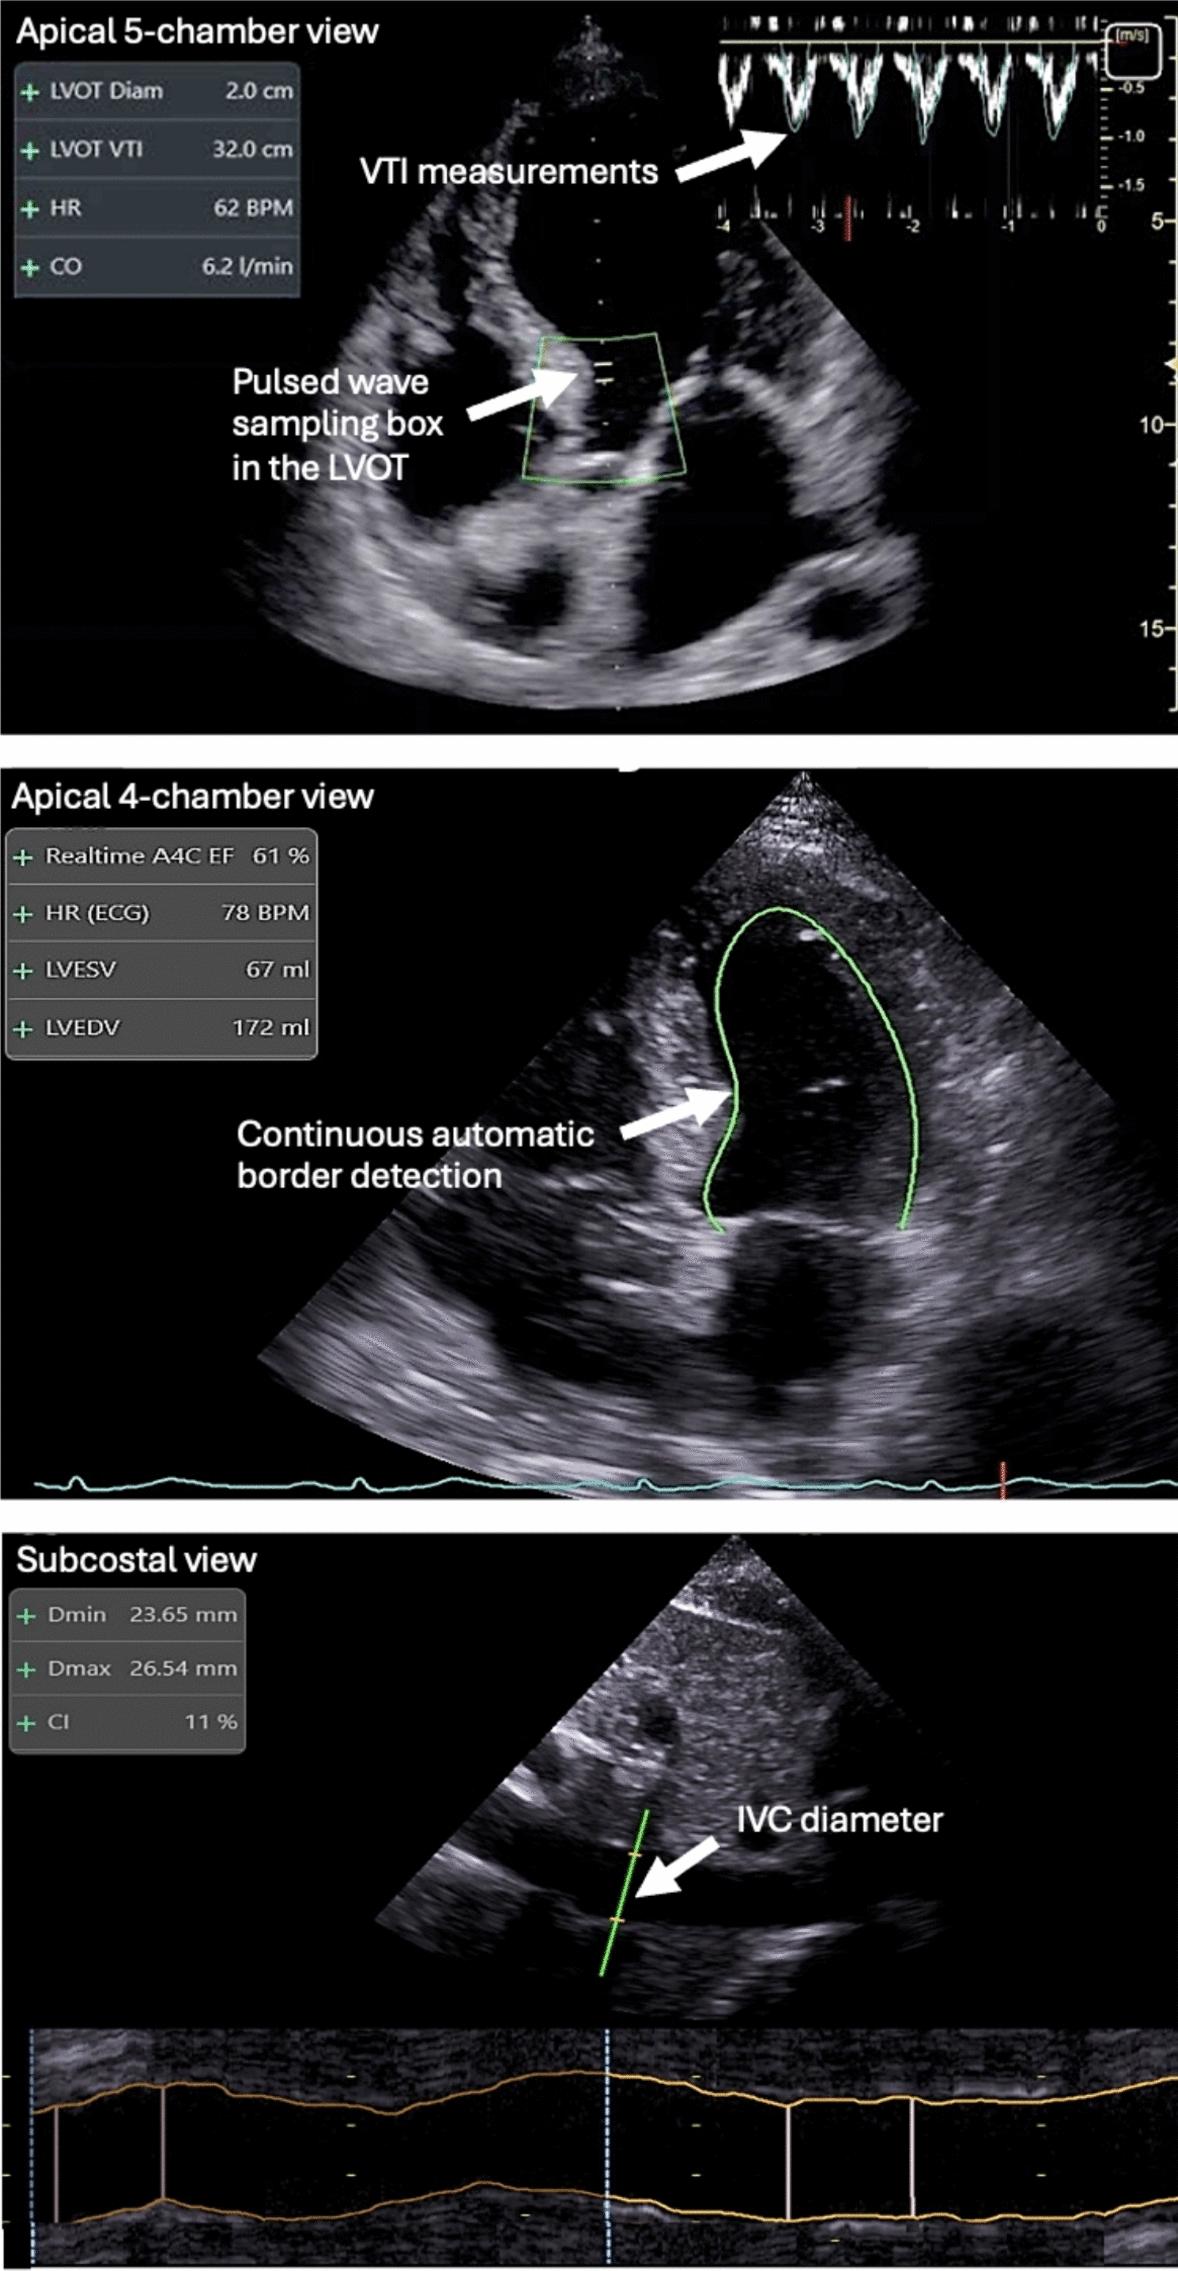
Examples of AI-enabled tools for echocardiography. Top: Automated Velocity Time Integral (VTI) measurement from an apical 5 chambers view, Middle: Automated Left Ventricular Ejection Fraction measurement from an apical 4 chambers view (Realtime A4C EF), Bottom: Automated measurement of the inferior vena cava (IVC) collapsibility index (CI) from a subcostal view. *LVOT* left ventricle outflow tract diameter, *Diam* diameter, *HR* heart rate, *CO* cardiac output, *LVESV* left ventricular end-systolic volume, *LVEDV* left ventricular end-diastolic volume, *Dmin* minimum diameter, *Dmax* maximum diameter
